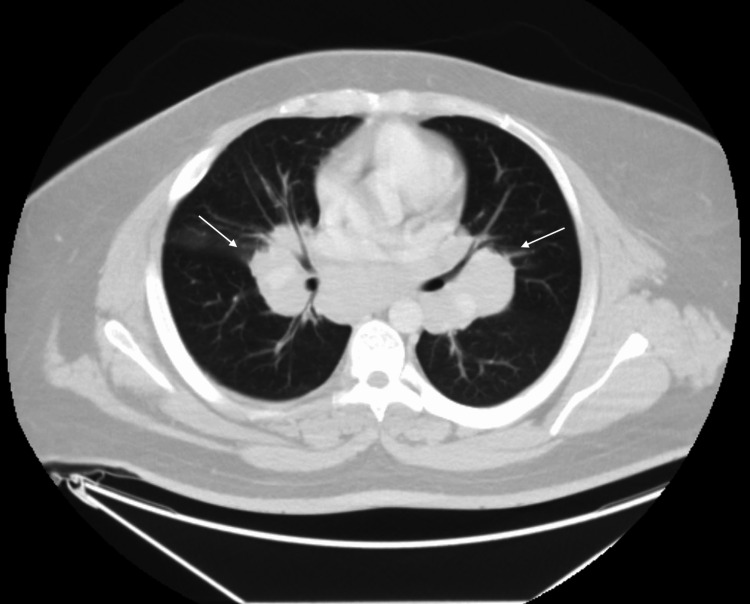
Chest computed tomography (CT) scan The image is demonstrating bulky diffuse mediastinal and hilar lymphadenopathy measuring up to 3.8 cm within the subcarinal space.
